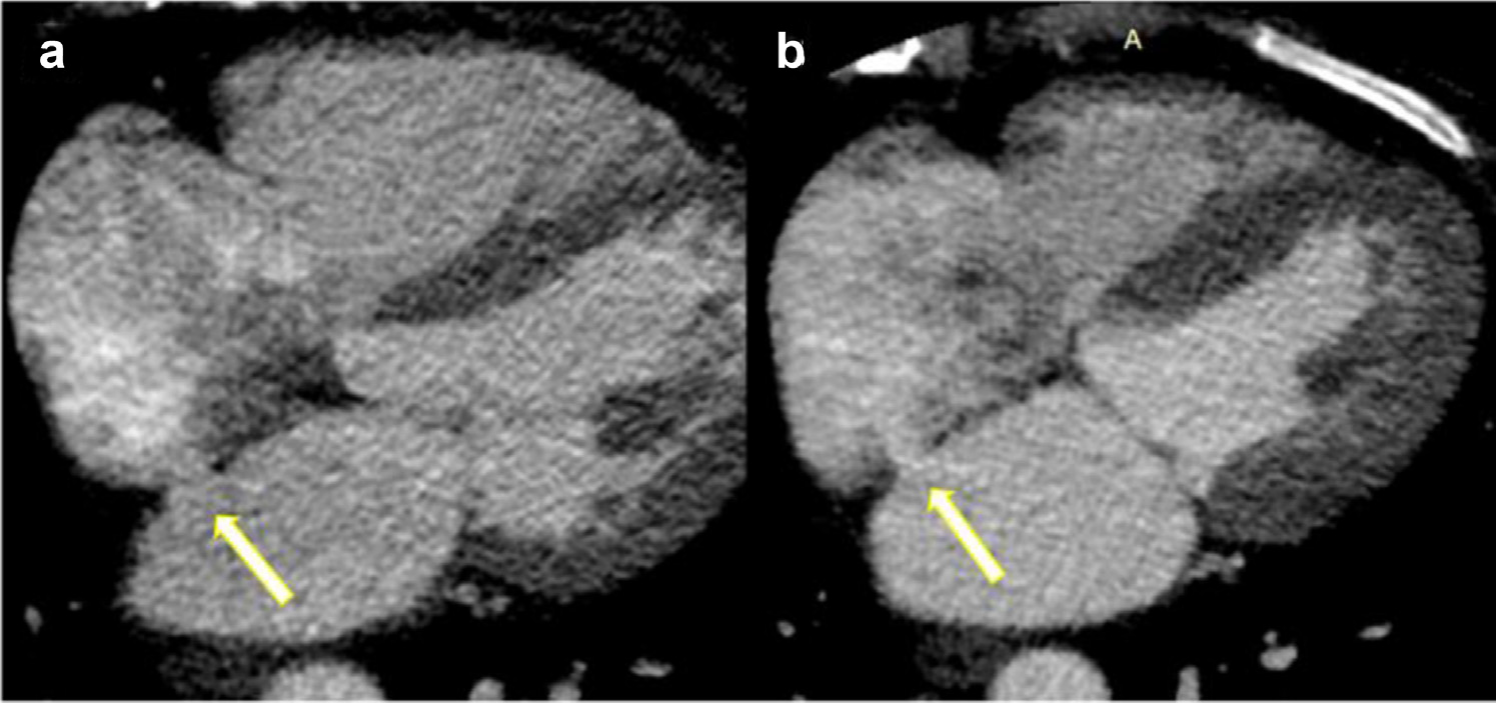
Representative images of cardiac computed tomography assessment showing shunt patency sustained through 6-month follow-up in a human subject. (a) Baseline, immediately postshunt creation. (b) Six-month follow-up.
